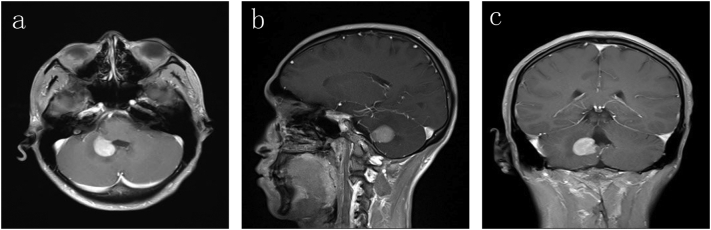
Magnetic resonance imaging showing a high enhancement signal in the right cerebellum.
